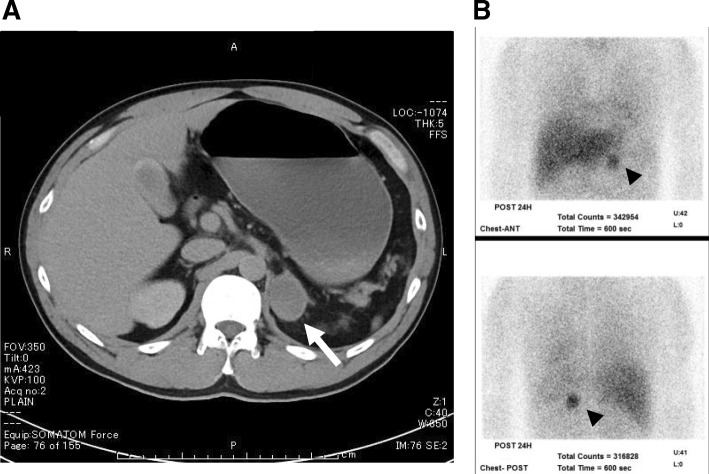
Images of abdominal computed tomography and functional scintigraphy using iodine-131 metaiodobenzylguanidine. a Abdominal computed tomography, the *arrow* indicates a left adrenal gland tumor. b Anterior (*upper*) and posterior (*lower*) reprojected images of iodine-131 metaiodobenzylguanidine scintigraphy, the *arrow head* indicates strong uptake in the region of the left adrenal gland
